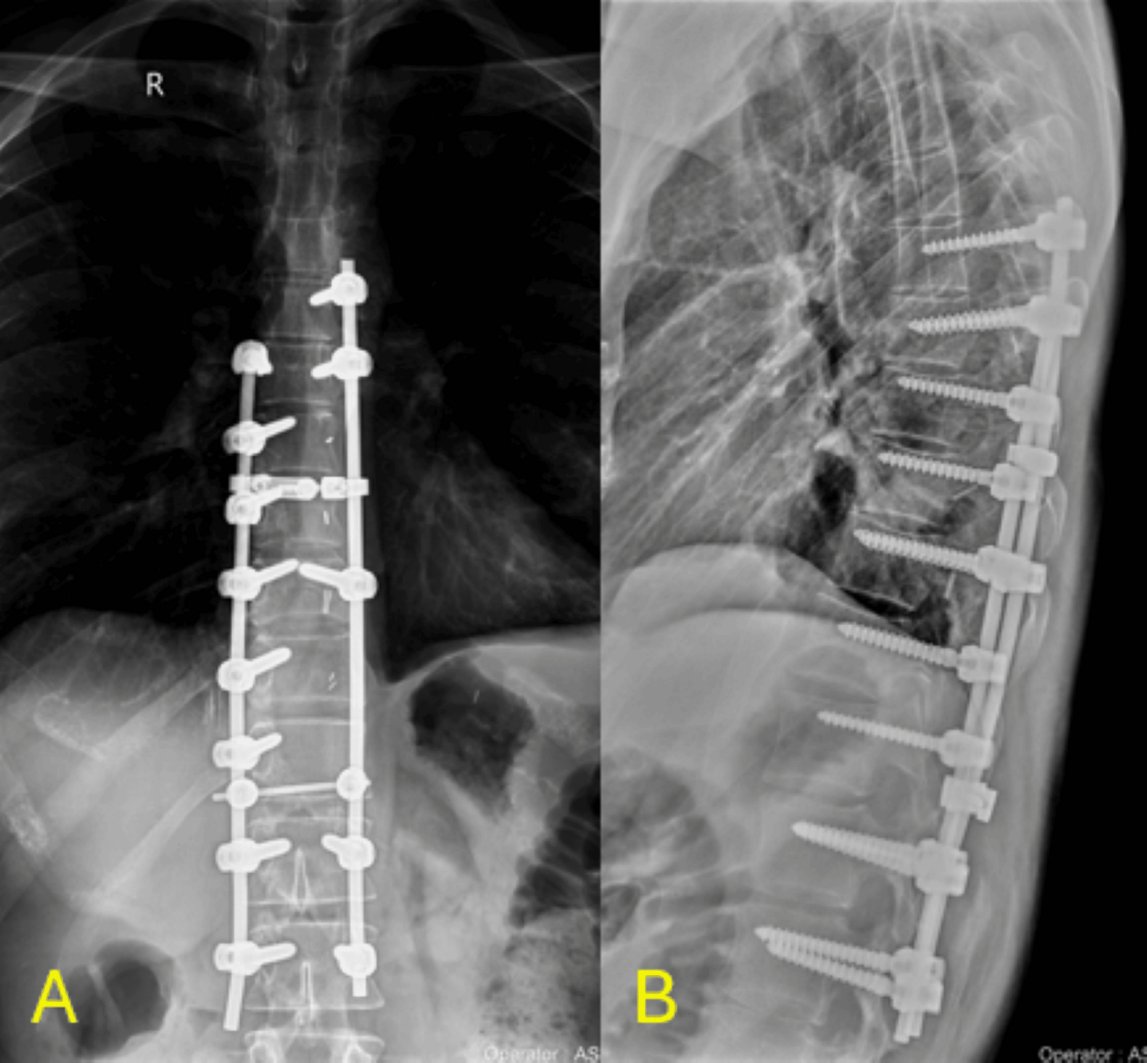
Follow-up X-ray eight months after surgery At the upper end of the construct, unilateral instrumentation was chosen to avoid creating a long lever arm between two screws, which could compromise the stability of the construct. By adding one additional screw distally, we ensured greater biomechanical stability and reduced the risk of hardware failure. 11A: Coronal X-ray showing spinal instrumentation at eight months post-surgically 11B: Sagittal X-ray showing spinal instrumentation at eighth months post-surgically
